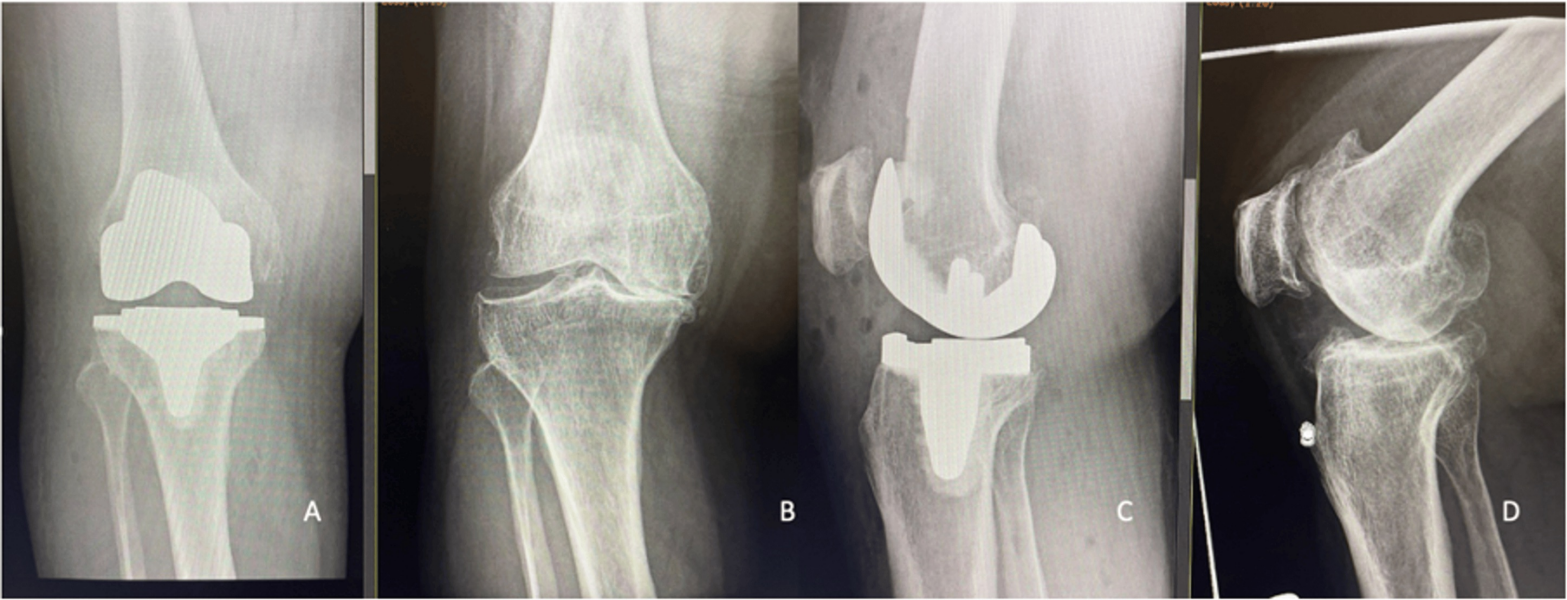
Preoperative (B and D) and postoperative (A and C) X-ray images of a patient with severe osteoarthritis
